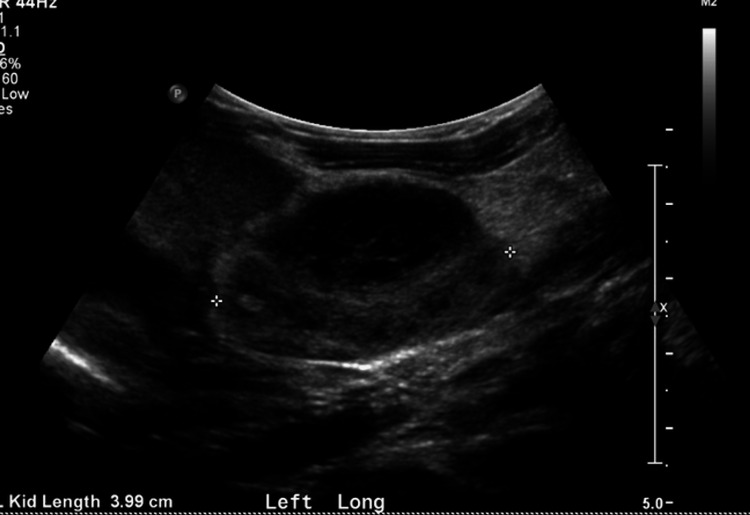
Ultrasound of the left kidney in the week after drain removal, demonstrating further decrease in the size of perinephric fluid collection and lack of hydronephrosis.
